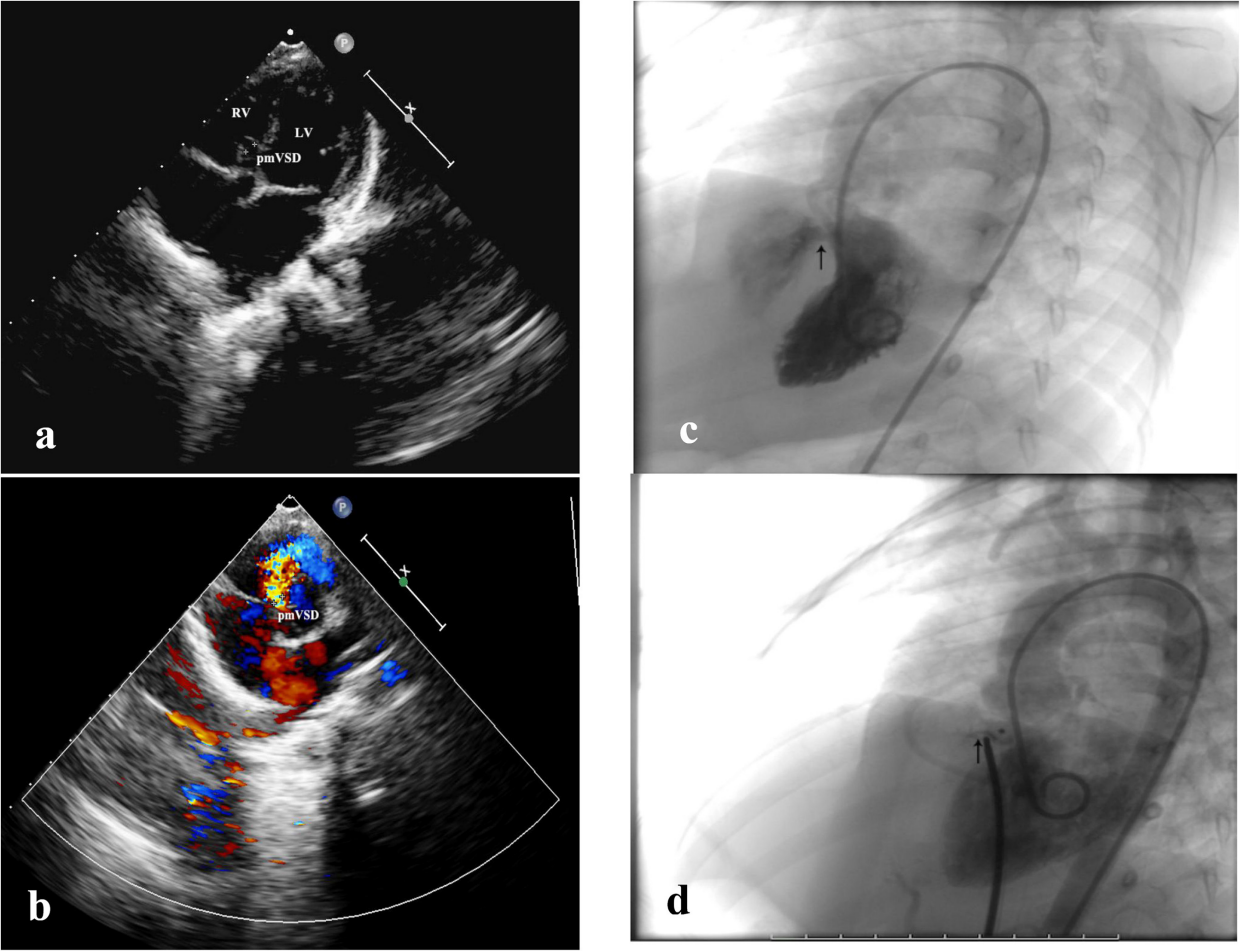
Echocardiography and DSA imaging. (a) Two-dimensional echocardiography showing pmVSD in the apical five-chambered view. (b) Color Doppler echocardiography showing pmVSD (left to right shunt) in the short-axis view. (c) DSA image showing left to right shunt through the pmVSD in the left anterior oblique position. (d) DSA image showing no residual shunt post transcatheter closure with MODO.
